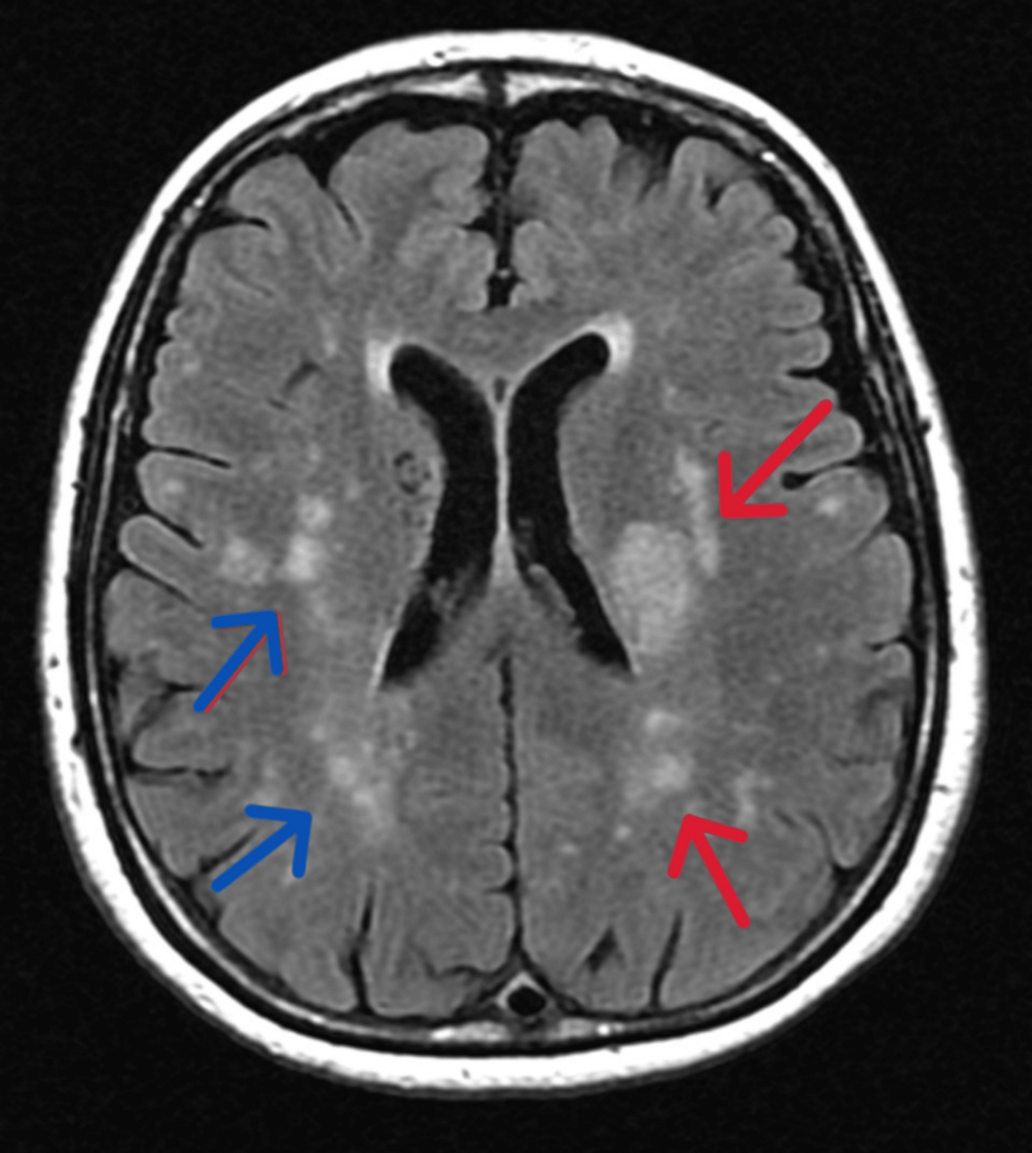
Axial view of cerebral MRI (T2 FLAIR). The red arrows (left hemisphere) show an area of restricted diffusion in the left corona radiata, posterior limb of the internal capsule, and small acute parietal lesion. The blue arrows (right hemisphere) reveal microvascular ischemic lesions MRI: magnetic resonance imaging; FLAIR: fluid-attenuated inversion recovery
